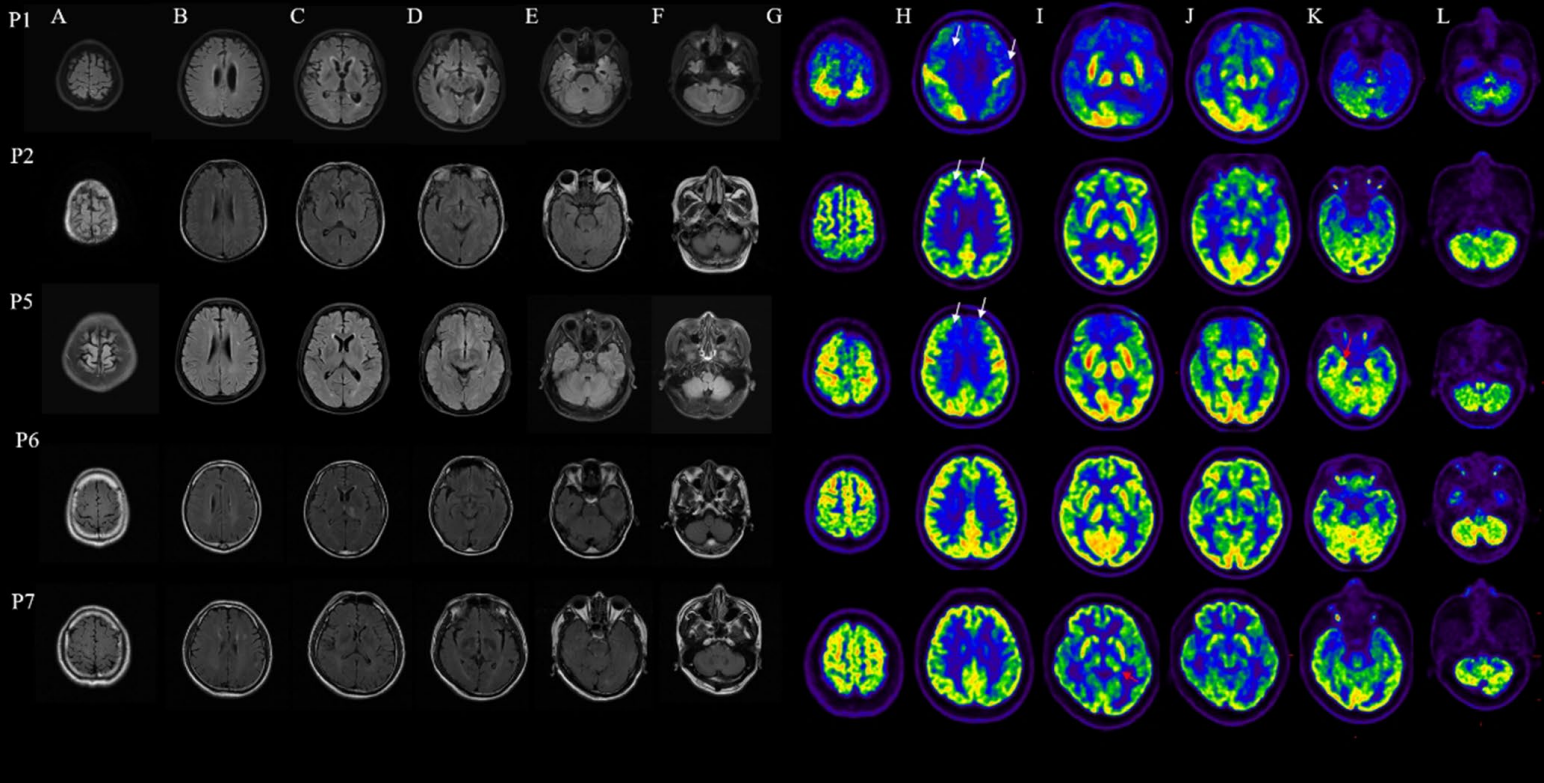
Brain MRI and ^18^F‐FDG PET/CT imaging in patients with anti‐AMPAR encephalitis. The MRI images of the brain are shown for Patients 1, 2, 5, 6, and 7 (A–F). Axial slices of ^18^F‐FDG PET/CT images from the vertex to the skull base are presented in panels (G–L). White arrows indicate regions of cortical hypometabolism, predominantly in the prefrontal cortex. In contrast, red arrows highlight areas of hypermetabolism. Patients 1, 2, and 5 exhibited marked prefrontal hypermetabolism (P1‐H, P2‐H, P5‐ H). Additionally, Patient 5 demonstrated hypermetabolism in the right temporal lobe (P5‐K), and Patient 7 showed increased metabolic activity in the left thalamus (P7‐K), consistent with focal neural activation or inflammation. ^18^F‐FDG PET/CT shows greater sensitivity than MRI.
